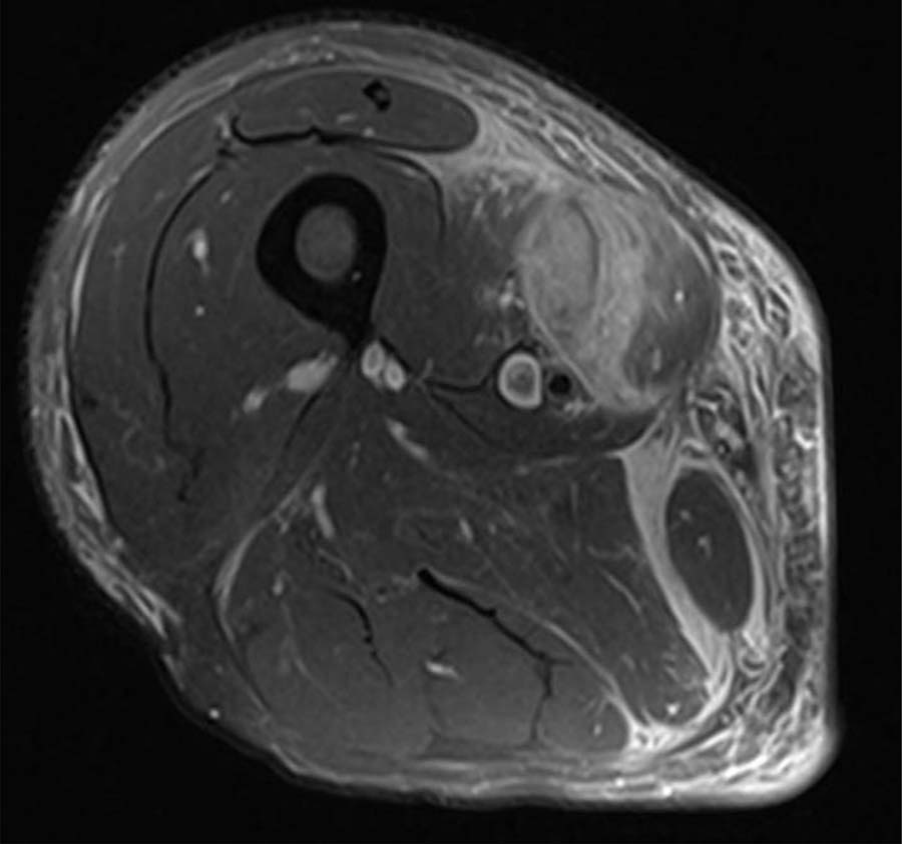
MRI axial view of right thigh region showing hematoma.
